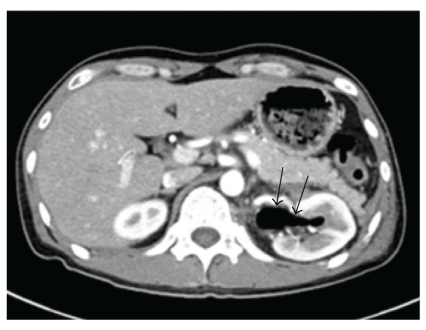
CT of the abdomen and pelvis. There is a gas shadow in the left renal pelvis (black arrows).
